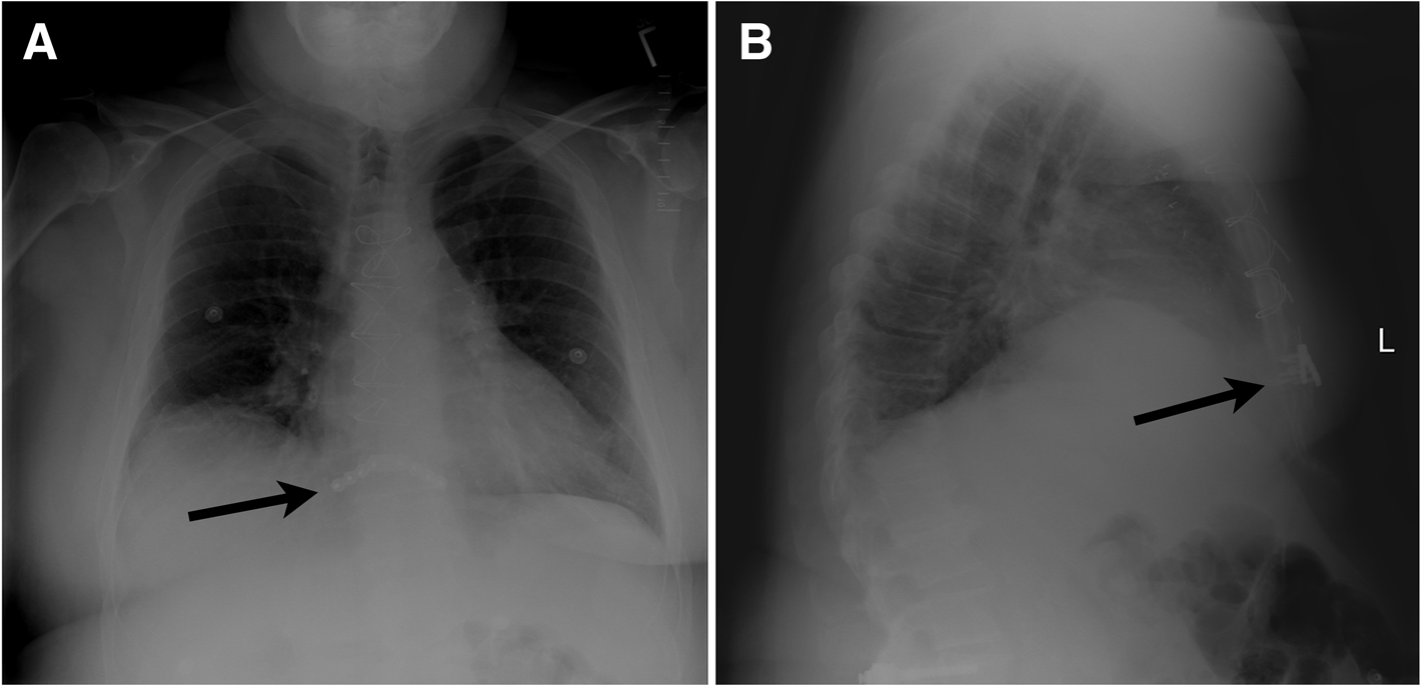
Plain chest radiographs taken 2 weeks after placement of a Synthes titanium plate (arrows). a Posterior-anterior view. b Lateral view
